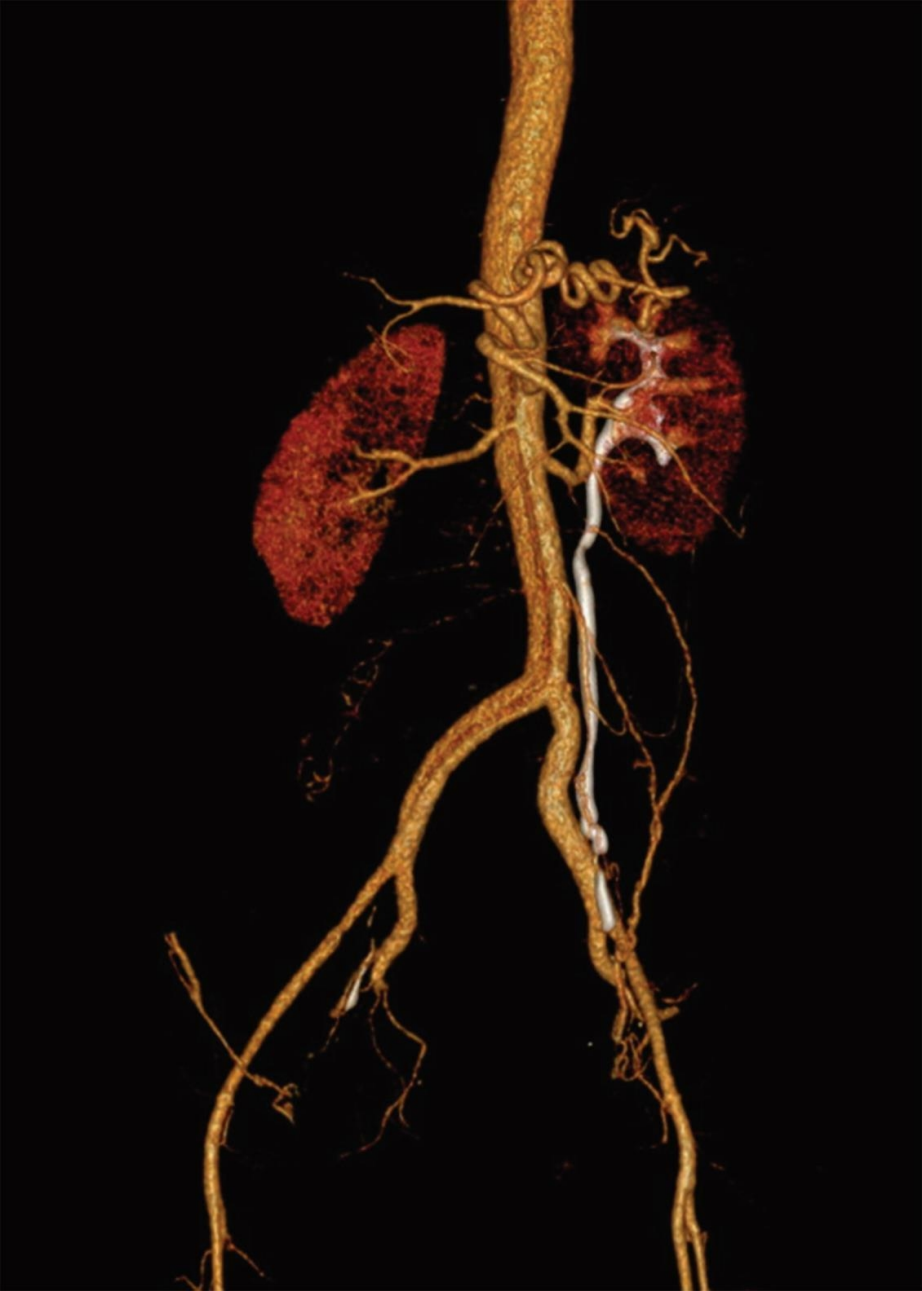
CT angiogram of abdomen/pelvis with and without contrast.
